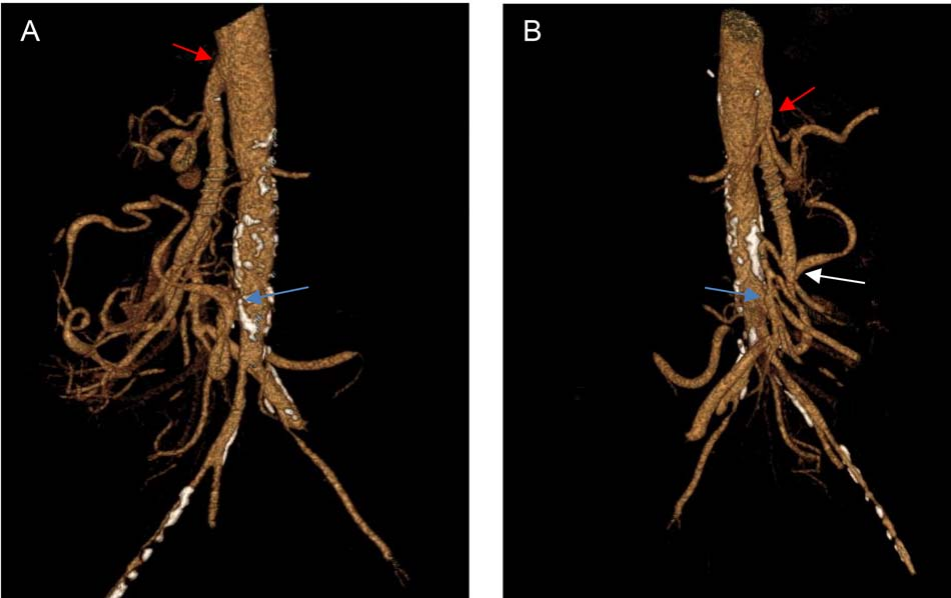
(A, B) Three-dimensional CT reconstruction at 1-year follow-up, showing the anastomosis of the CT (red arrow), the anastomosis of the SMA (white arrow) and the reimplantation of the IMA (yellow arrow) with excellent graft patency.
